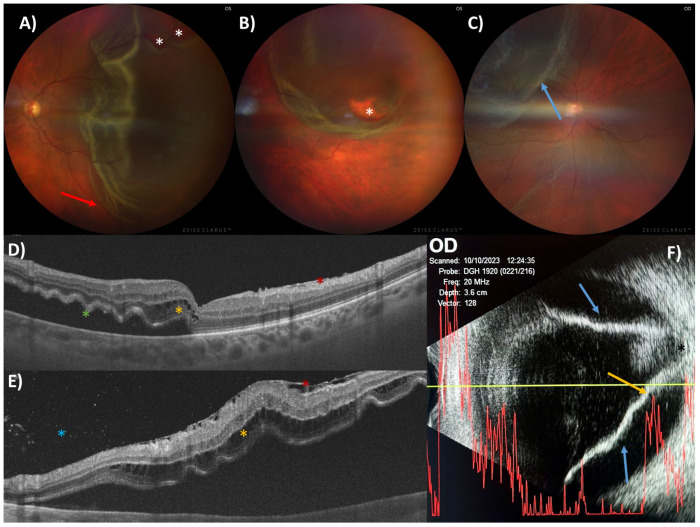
Alterations of retinal structures in patients with RRD. Color fundus photography (A–C), spectral-domain optical coherence tomography (SD-OCT) (D,E), and A- and B-mode ocular ultrasound (F) of several patients with RD. (A,E) correspond to the same patient. Fundus photography (A–C) enables visualization of retinal tears (indicated by the white asterisk) and the presence of retinal folds (red arrow), which may suggest that the lesion has been present for some time. (C) corresponds to a reRD showing a paler shade of the detached layers (indicated by the blue arrow). SD-OCT (D,E) shows multiple intraretinal cysts (yellow asterisk), epiretinal membrane (red asterisk), retinal neurosensory folds (green asterisk), Shaffer’s sign (blue asterisk), and subfoveal neuroretinal detachment. B-mode ultrasound (F) showing a funnel-shaped RD caused by a retinal detachment that respects the papillary zone (blue arrows). A-mode ultrasound shows a peak of hyperechogenicity corresponding to the detached neurosensory retina and a second peak of hyperechogenicity corresponding to the RPE (yellow arrow).
